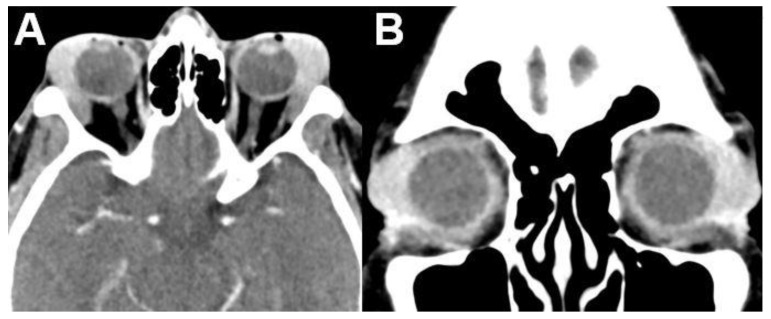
Mantle cell lymphoma.* CT of a 60–year-old female patient with mantle cell lymphoma showing bilateral involvement of the lacrimal gland. On axial (A) and coronal (B) images, lymphoma appears as a well-defined, homogenously enhancing mass of the bilateral lacrimal glands.
